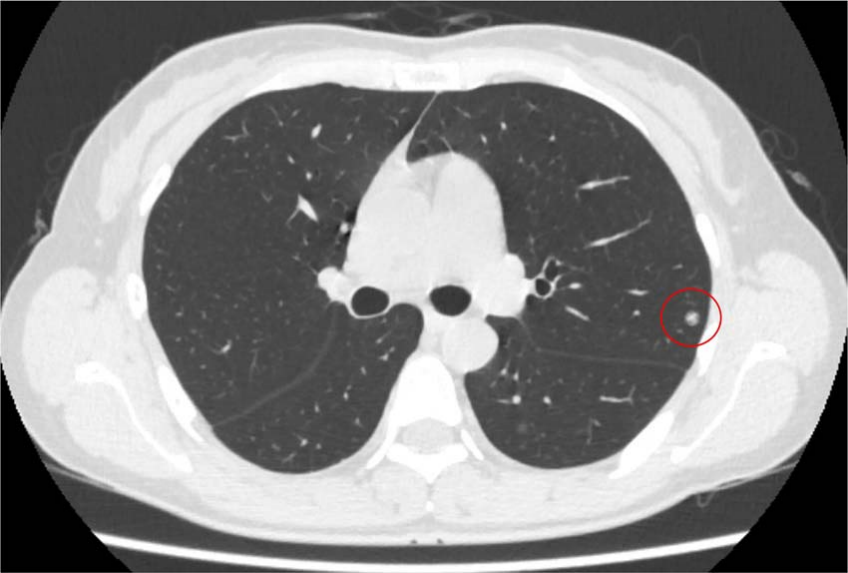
Computed tomography revealed a solitary pulmonary nodule (circle) in the left upper lobe 6 months after uterine surgery.
